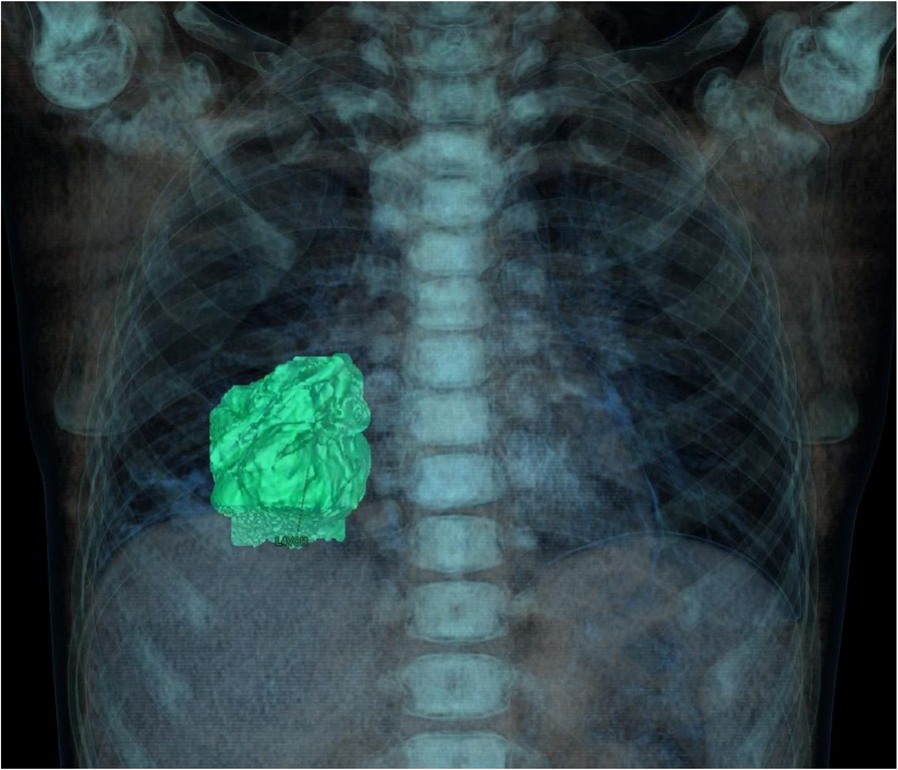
Chest 3D CT scan, volume rendering technique (VRT), evaluating the tumor mass
